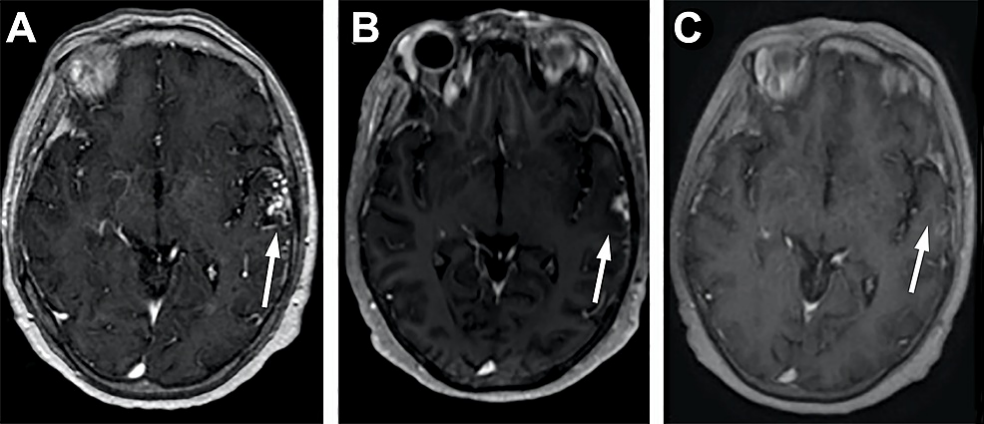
Serial T1-weighted post-contrast magnetic resonance imaging (MRI) scans indicating original presentation of left temporal arteriovenous malformation (AVM) and involution of lesion after stereotactic radiosurgery (SRS) (arrows) (A) MRI of the patient at the time of presentation for AVM. (B) MRI from 15 months post-SRS showing decreased size of AVM. (C) MRI from four years post-SRS with the resolution of AVM.
